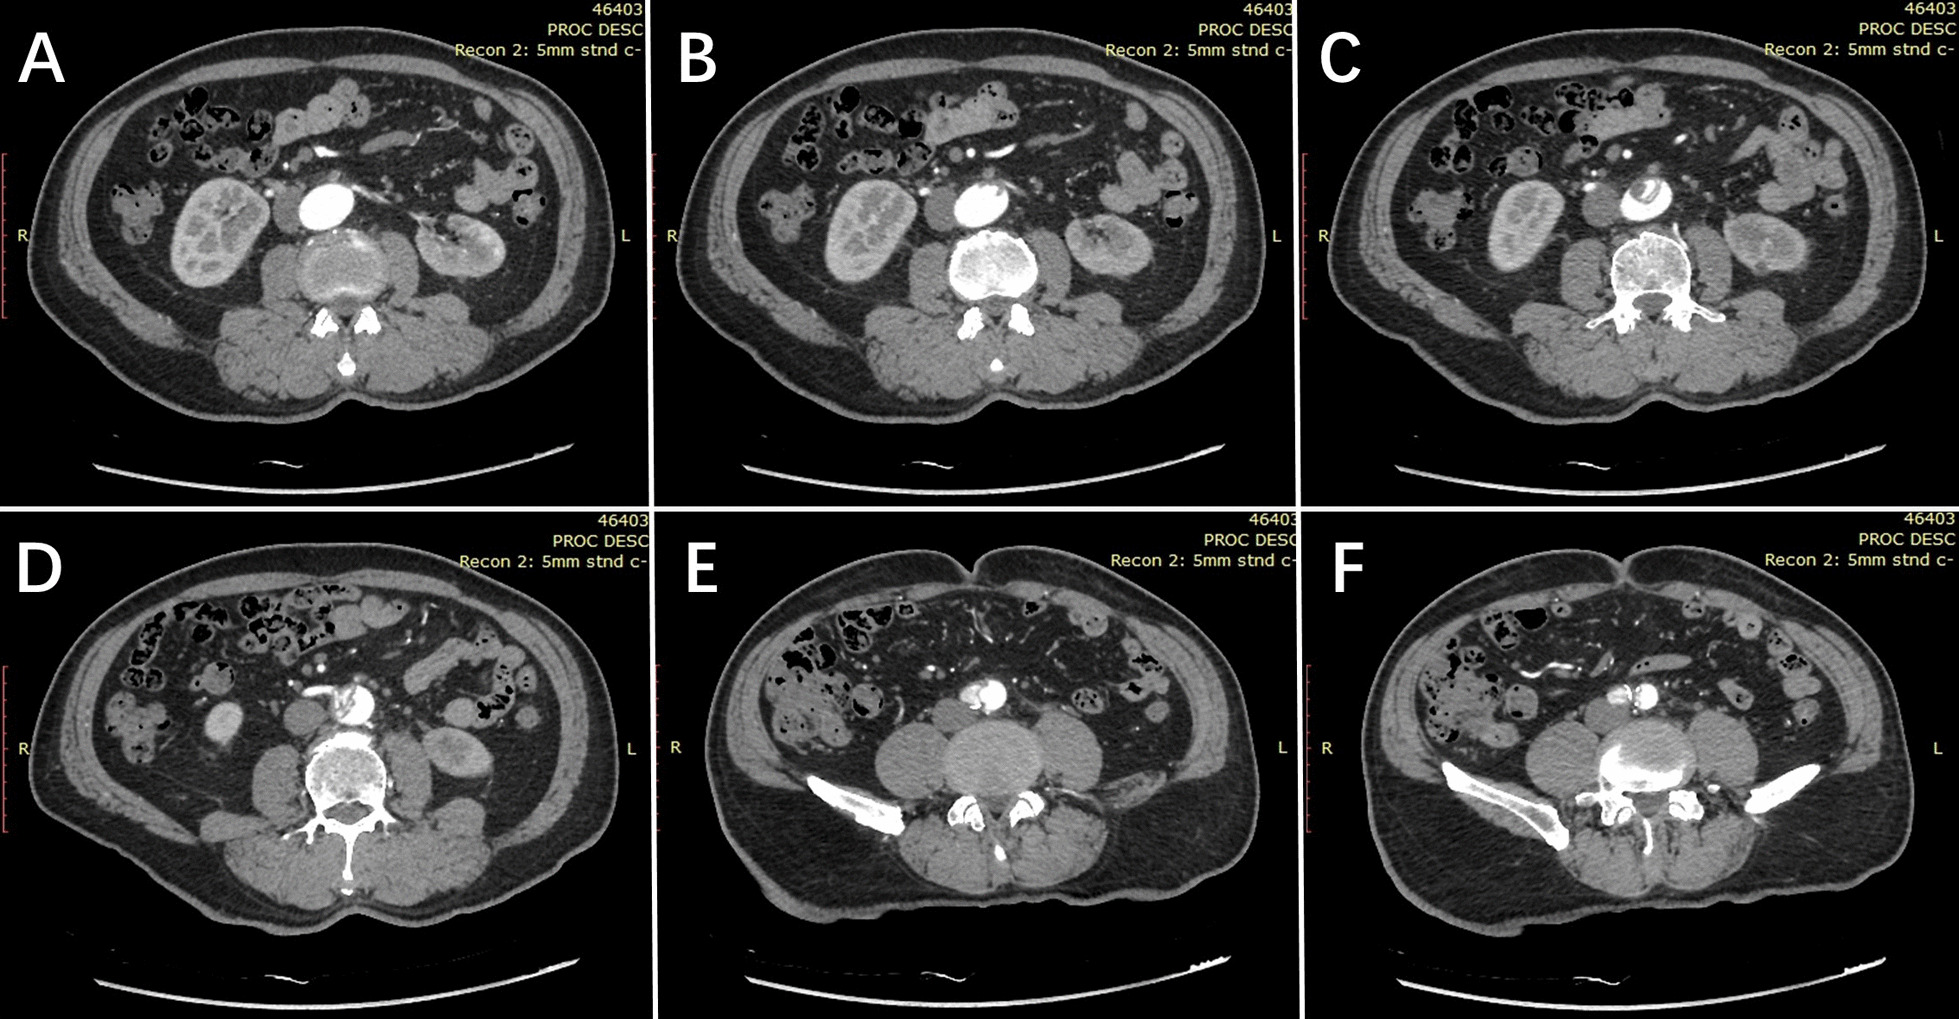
Preoperative axial-view surveillance computed tomography scans. A Normal aorta above the left renal ostia. B The proximal end of abdominal aortic dissection beneath the left renal ostia. C The middle part of the abdominal aortic dissection. D Inferior mesenteric artery supplied by the false aortic lumen. E The aorta above the bifurcation. F Right iliac artery supplied by false aortic lumen
